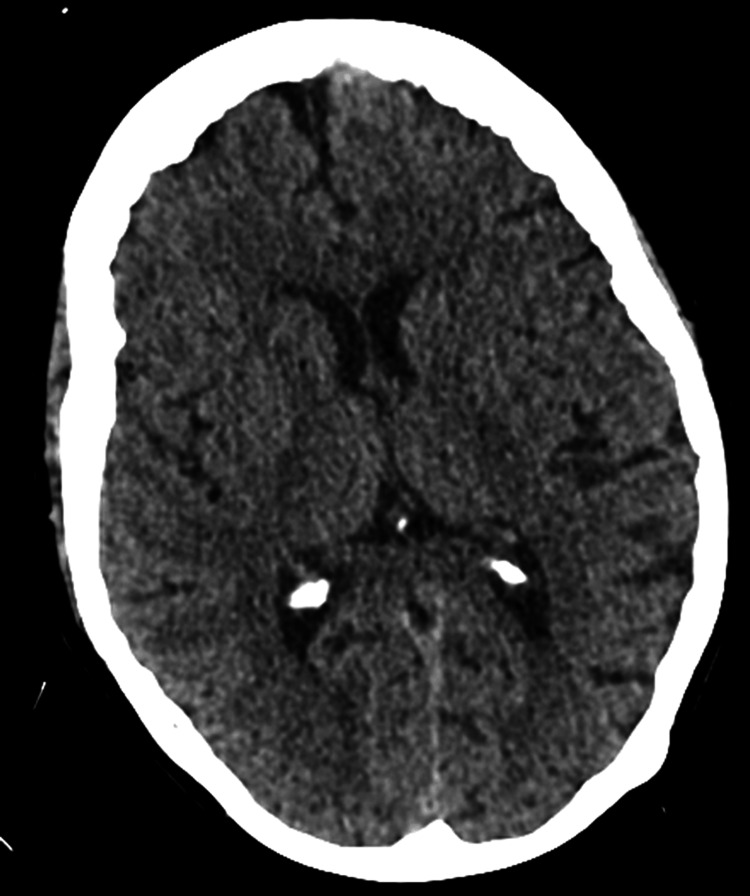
Patient's CT head scan demonstrating no acute abnormality with normal parenchymal volume and preserved grey-white matter differentiation CT: computed tomography
